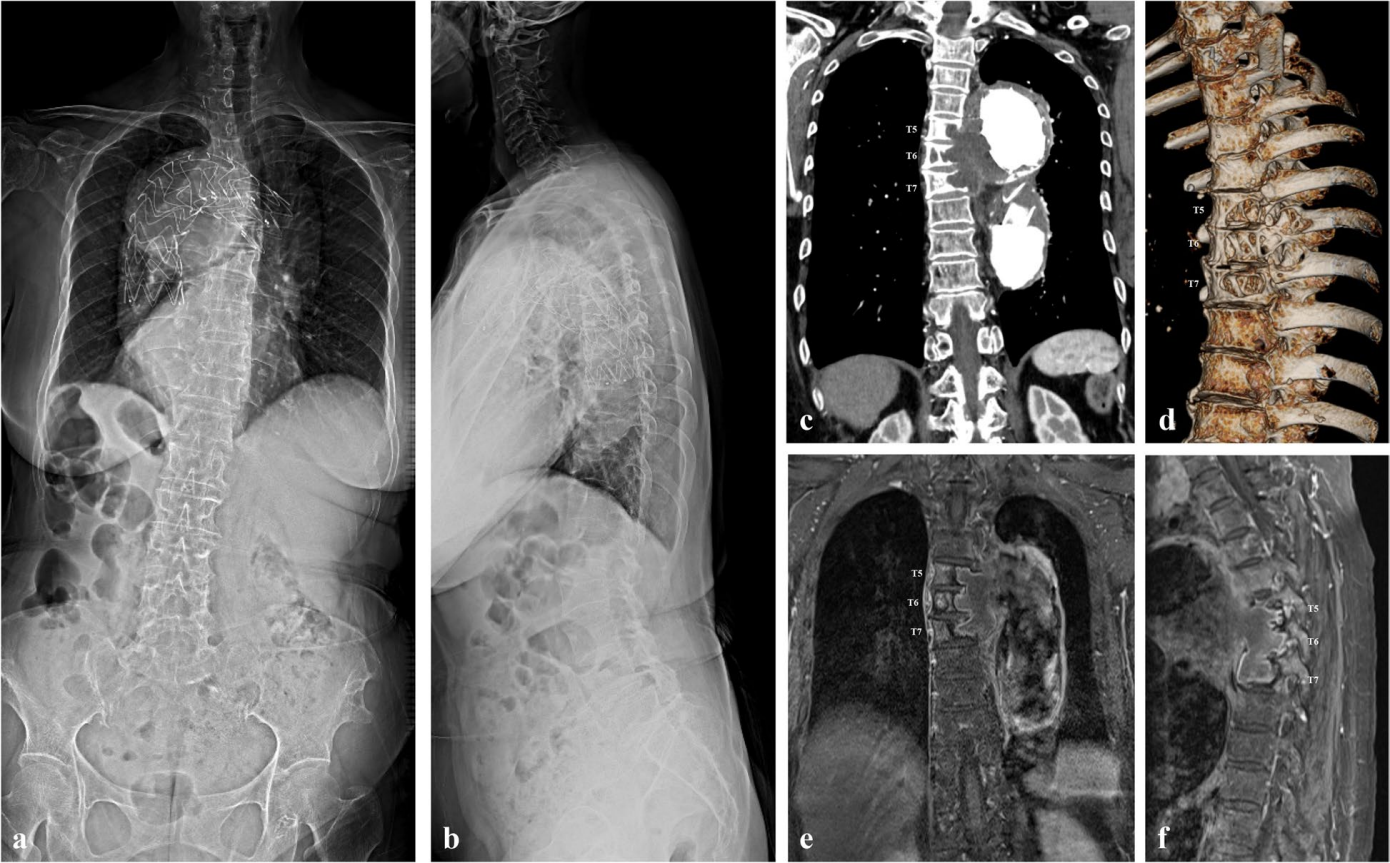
Initial presentation of this case. a and b, Plain radiographs revealing the implanted stent graft at the proximal descending thoracic aorta. c and d, Computed tomography of the aorta and thoracic spine show bony erosion into the T5–T7 vertebral bodies. e and f, Magnetic resonance imaging of the thoracic spine confirms a perianeurysmal outpouching lesion eroding into the T5–T7 vertebral bodies due to pulsating pressure
